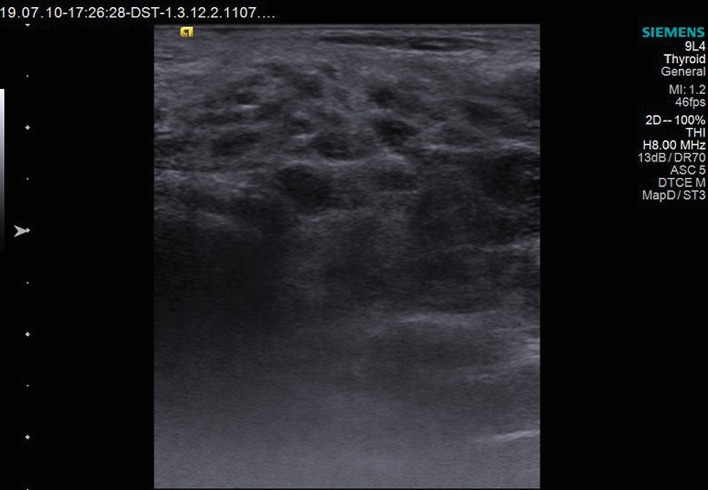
B-mode ultrasonography of the parotid gland.
